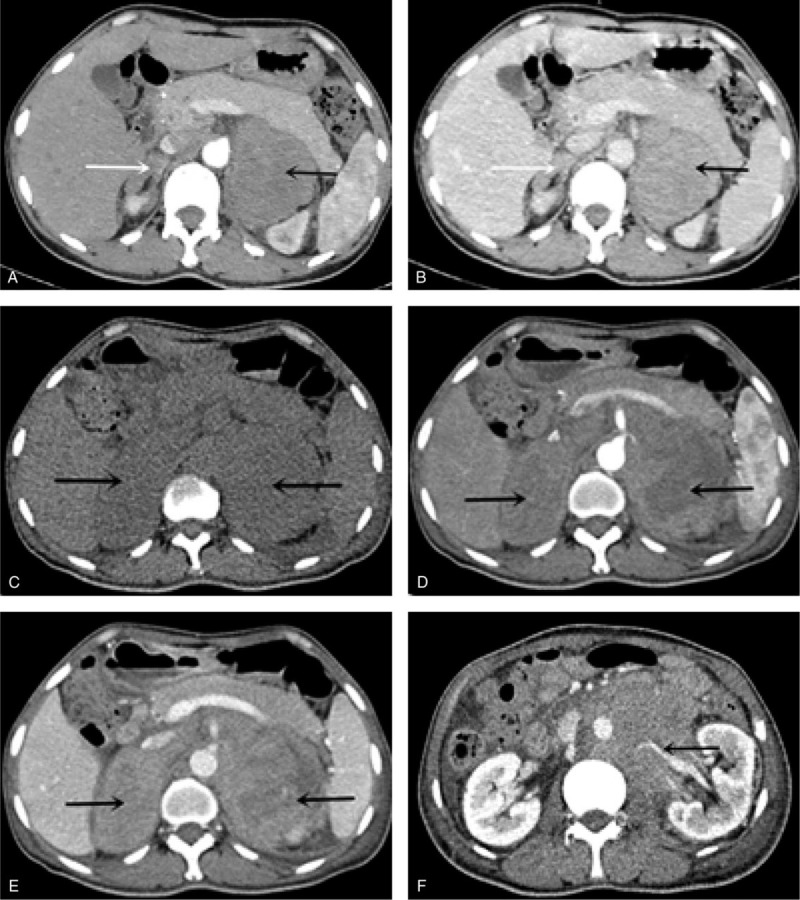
Fast-growing image features of the patient with Primary adrenal lymphoma: compared the first abdominal CT scan (A-B arterial and parenchymal phase) with the second adrenal CT scan (C-E unenhanced, arterial and parenchymal phase), it demonstrated further enlargement of bilateral adrenal masses along with visible bleeding necrosis, local infiltration of the renal artery and retroperitoneal lymphadenopathy.
